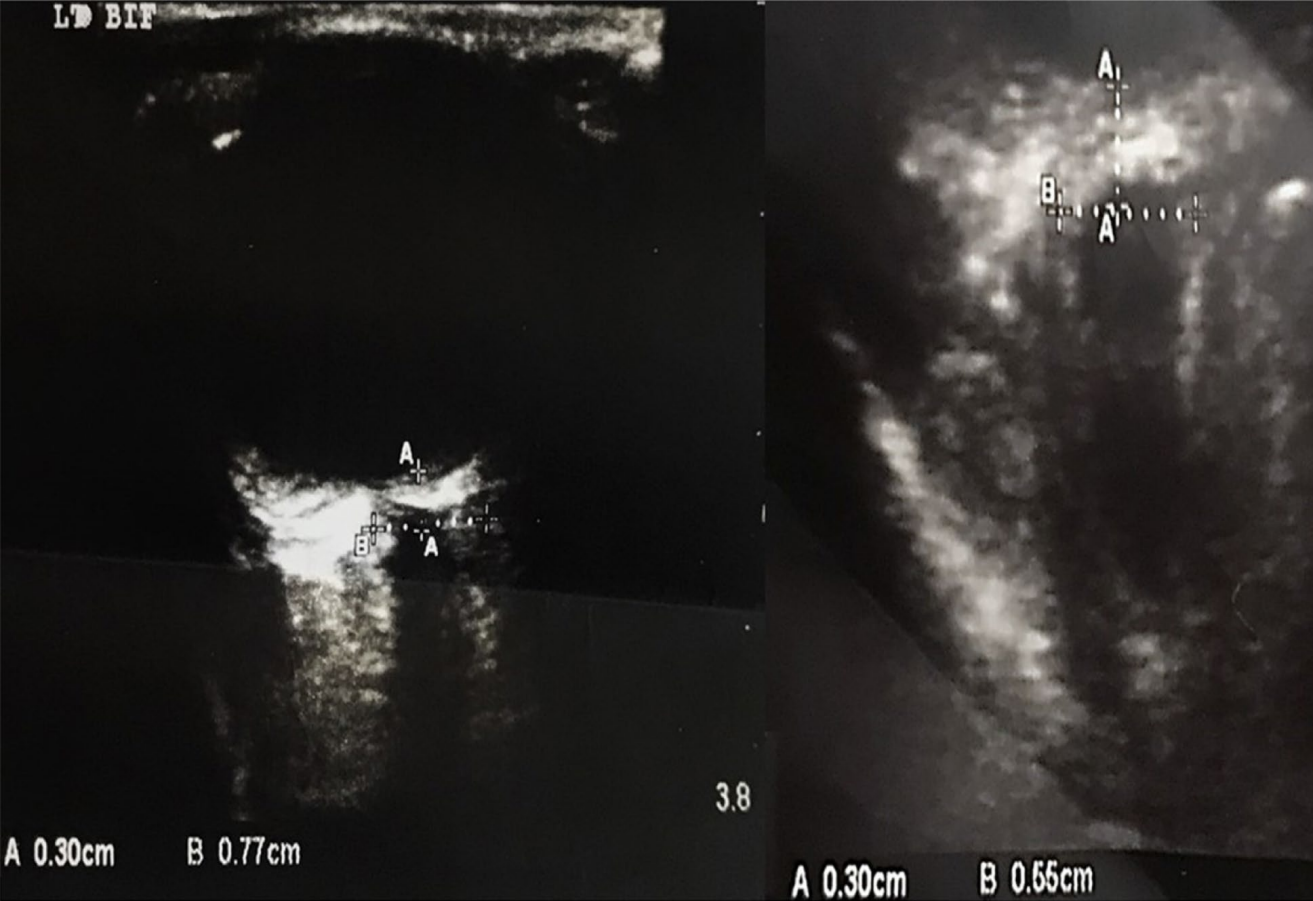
Ultrasound of optic nerve sheath using an electronic caliper along the axis of the optic nerve
